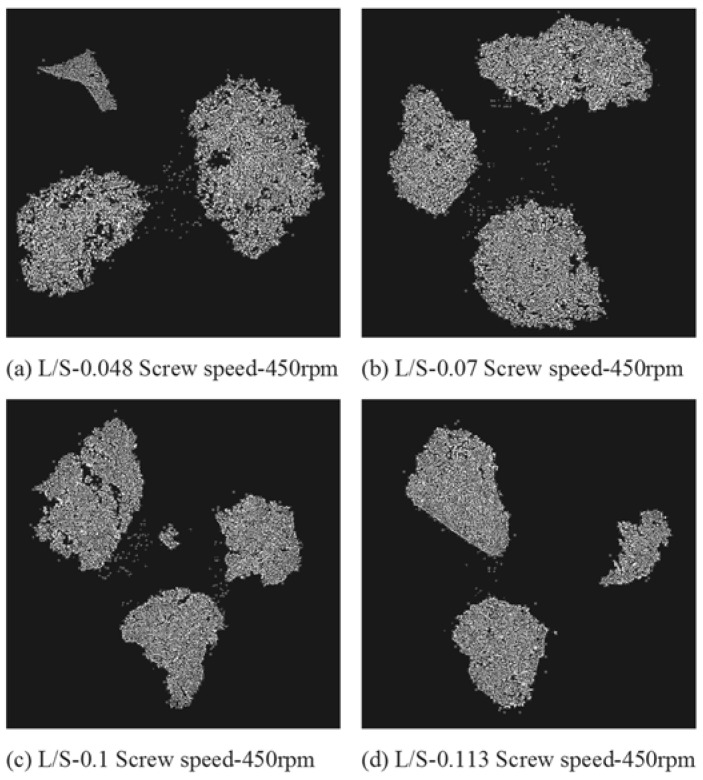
X-ray tomographic images of granules produced using Pharmatose 200M at varying L/S (screw speed, 450 rpm).
